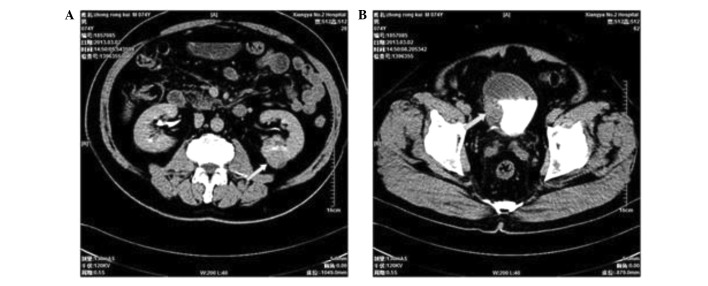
(A) Contrast-enhanced CT of the abdomen and pelvis of the patient showed an enhancing mass (arrow) in the left of the kidney. (B) Delayed-phase CT of the pelvis of the patient showed a mass of homogeneous low attenuation in the right and front walls of the bladder (arrow). CT, computerised tomography.
